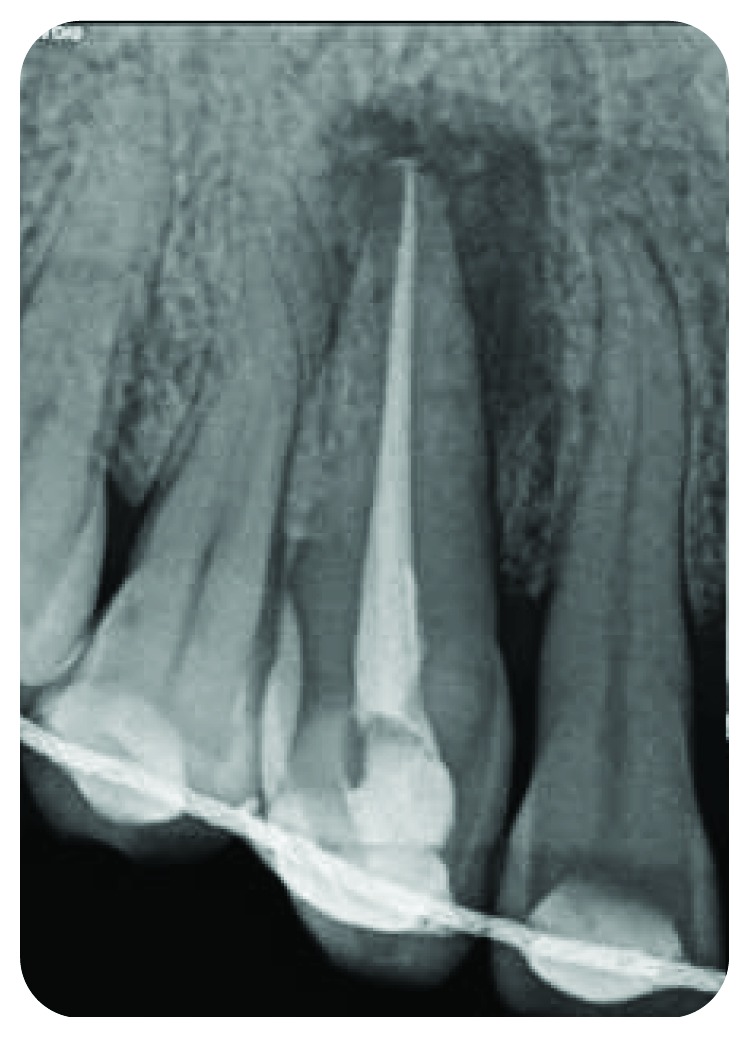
Six months' recall radiograph.
